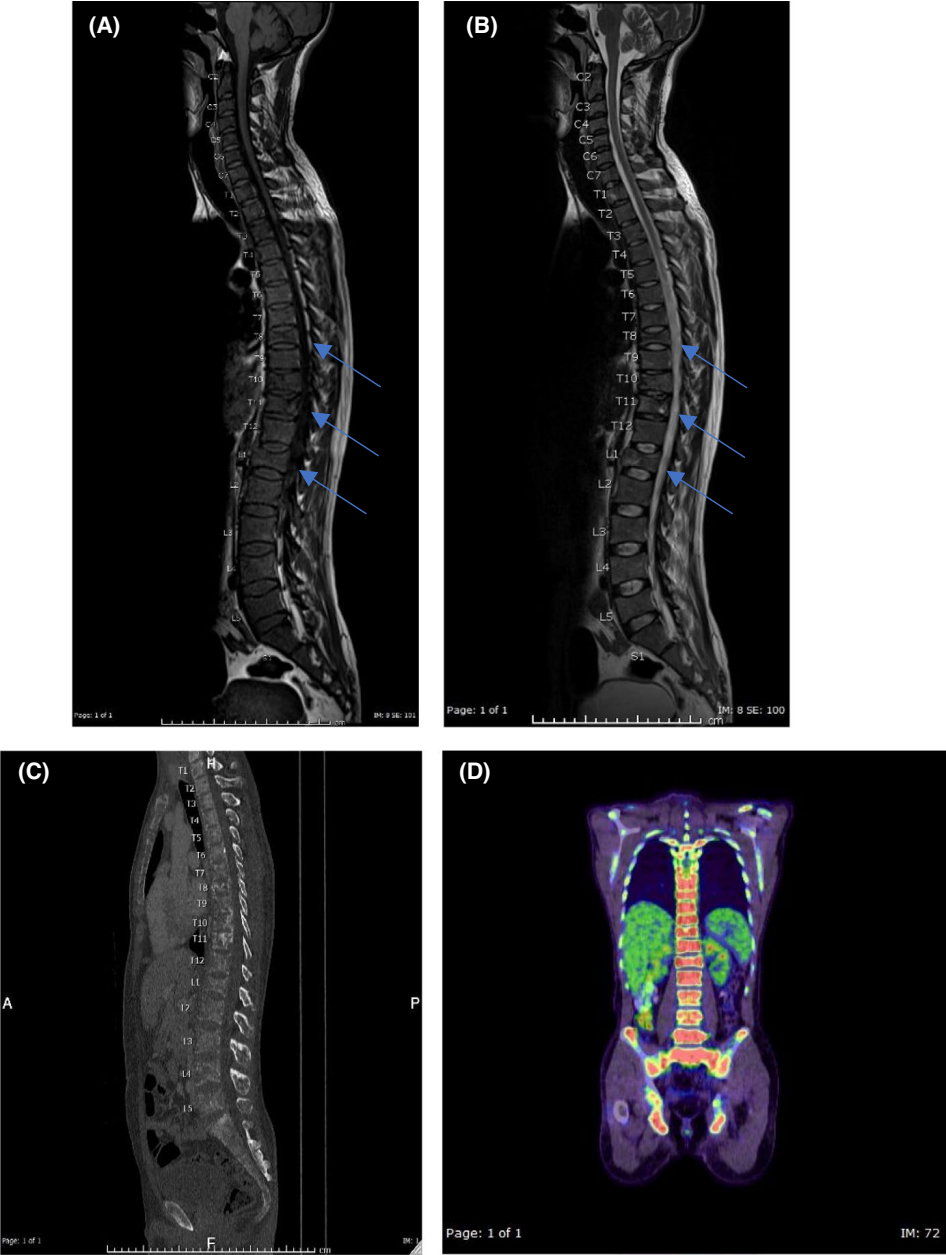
(A) Sagittal T1‐WI of the whole spine and B) Sagittal T2‐ WI of whole spine show heterogenous appearance of bone marrow of the spine with relative diffuse low signal intensity of bone marrow in T1 ‐WI and reduced height of multiple vertebral bodies affecting mainly T8, T11 and L1 (Arrows in A &B). (C) Sagittal reformatted Computed Tomography image of the Thoracic and lumbosacral spine in bone window setting show diffuse mainly lytic bony lesions involving the scanned spine and sternum with redemostration of reduced height of T8, T11 and L1. (D) Coronal color‐coded PET‐Scan image of the whole body show increased tracer uptake in the whole spine and the visualized parts of the pelvic bones (red color in D)
